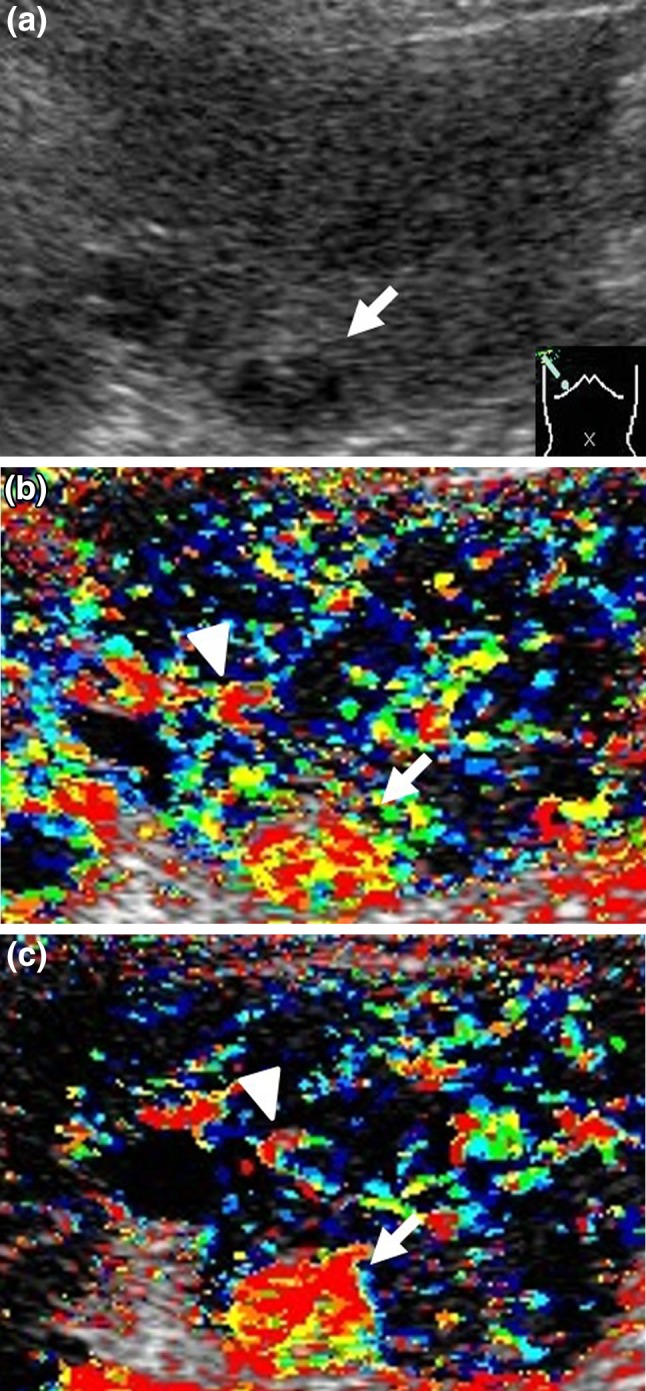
Clinical example of MT (−) group. The patient was a 71-year-old male with chronic hepatitis C virus. Sorafenib administration (400 mg/day) was started for advanced hepatocellular carcinoma (HCC). a Gray-scale ultrasonography showed a low echoic tumor measuring 16 mm in diameter in S6 (*arrow*). This tumor was established as a target lesion. b The color mapping image before treatment showed primarily* red* or* yellow* in the tumor (*arrow*). A large artery near this tumor was regarded as the reference point (*arrow head*). c The color mapping image 2 weeks after treatment showed primarily red in the tumor (*arrow*). The same artery before treatment was regarded as the reference point (*arrow head*)
